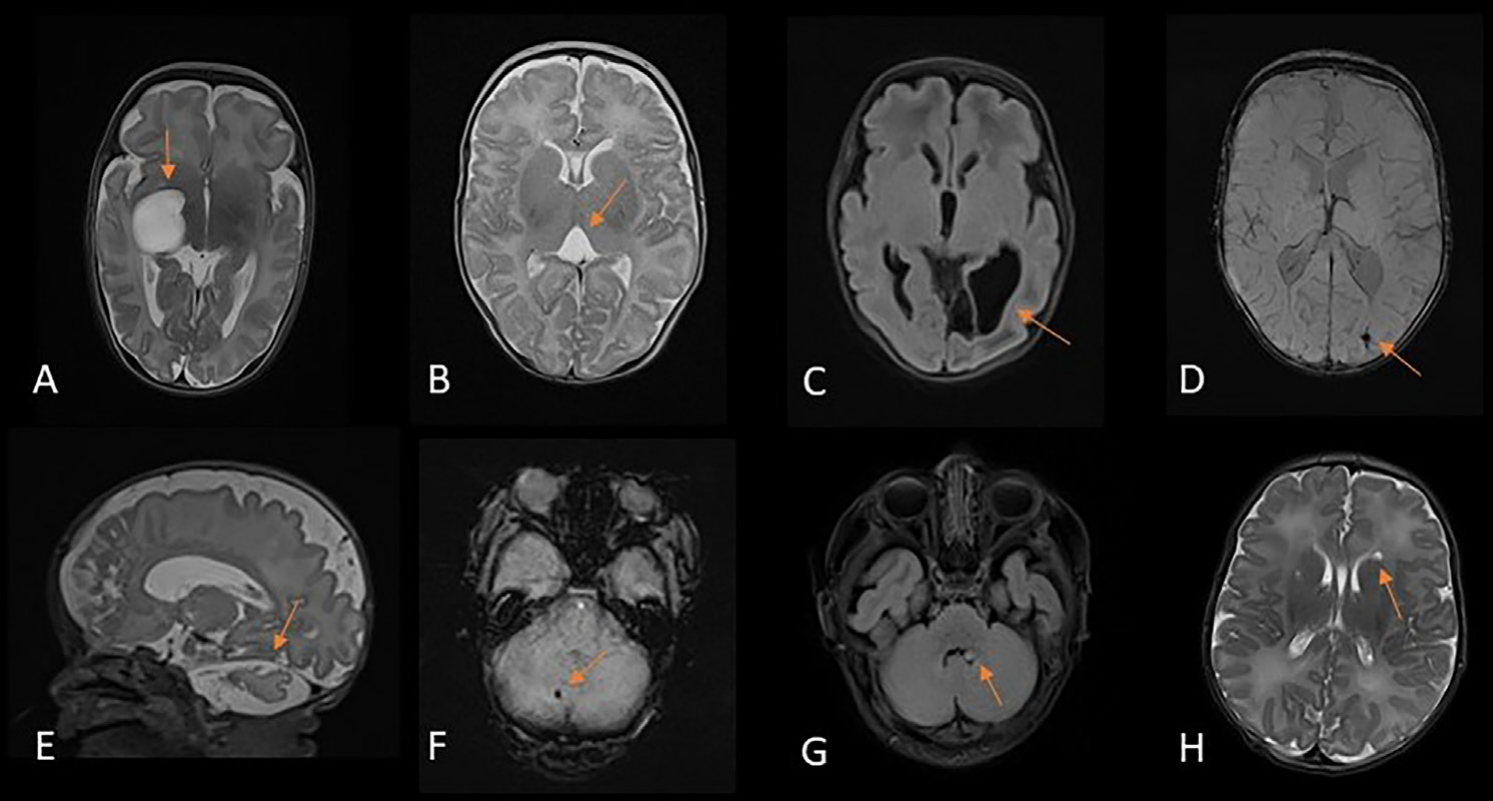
Incidental findings. (A) T2-weighted image of an infant aged 27^+4^ weeks, scanned at 42^+1^ weeks with a large middle cranial fossa cyst. (B) T2-weighted image of an infant aged 28^+5^ weeks, scanned at 40^+1^ weeks showing a cavum velum interpositum. (C) T2 BLADE of an infant aged 32^+3^ weeks, scanned at 36^+2^ weeks with cystic change in the left occipital lobe. (D) SWI of an infant aged 27^+2^ weeks, scanned at 42^+0^ weeks, demonstrating cystic change in the left occipital lobe. (E) T2 image of an infant aged 29^+3^ weeks, scanned at 40^+4^ weeks, demonstrating increased subarachnoid space and suspected pontine cerebellar hypoplasia. (F) SWI of an infant aged 31^+1^ weeks, scanned at 37^+6^ weeks, demonstrating a focus of abnormality between the right cerebellar hemisphere and vermis due to developmental venous anomaly. (G) T2 BLADE of an infant aged 40^+1^ weeks, scanned at 43^+3^ weeks, showing a 4 mm cyst on the floor of the fourth ventricle. (H) T2 BLADE of an infant aged 39^+1^ weeks, scanned at 43^+0^ weeks, showing a left connatal cyst.
